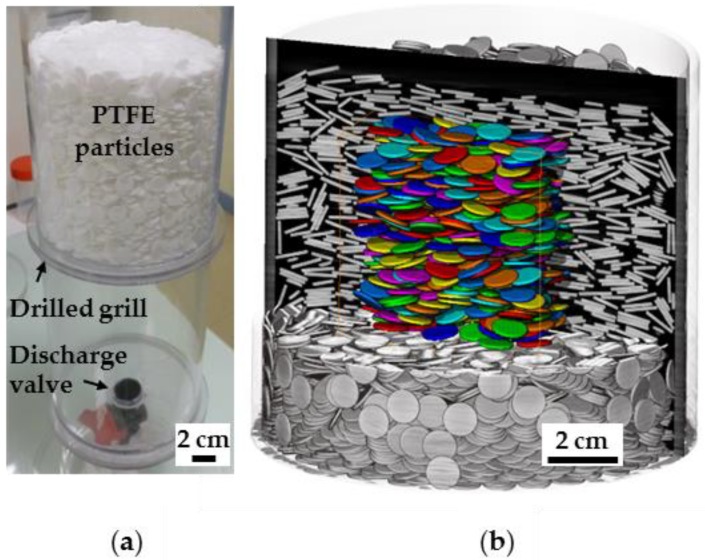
Experimental setup for packing of polytetrafluoroethylene (PTFE) disks. (a) Cylindrical column for sedimentation of 10,000 disks in different fluids. Disks are settled in the volume located above a flat drilled grill while evacuation of fluids is achieved through a discharge valve located at the bottom of the column; (b) X-ray microtomography analysis and extraction of individual particle orientation in a sub-volume of the experimental packing.
